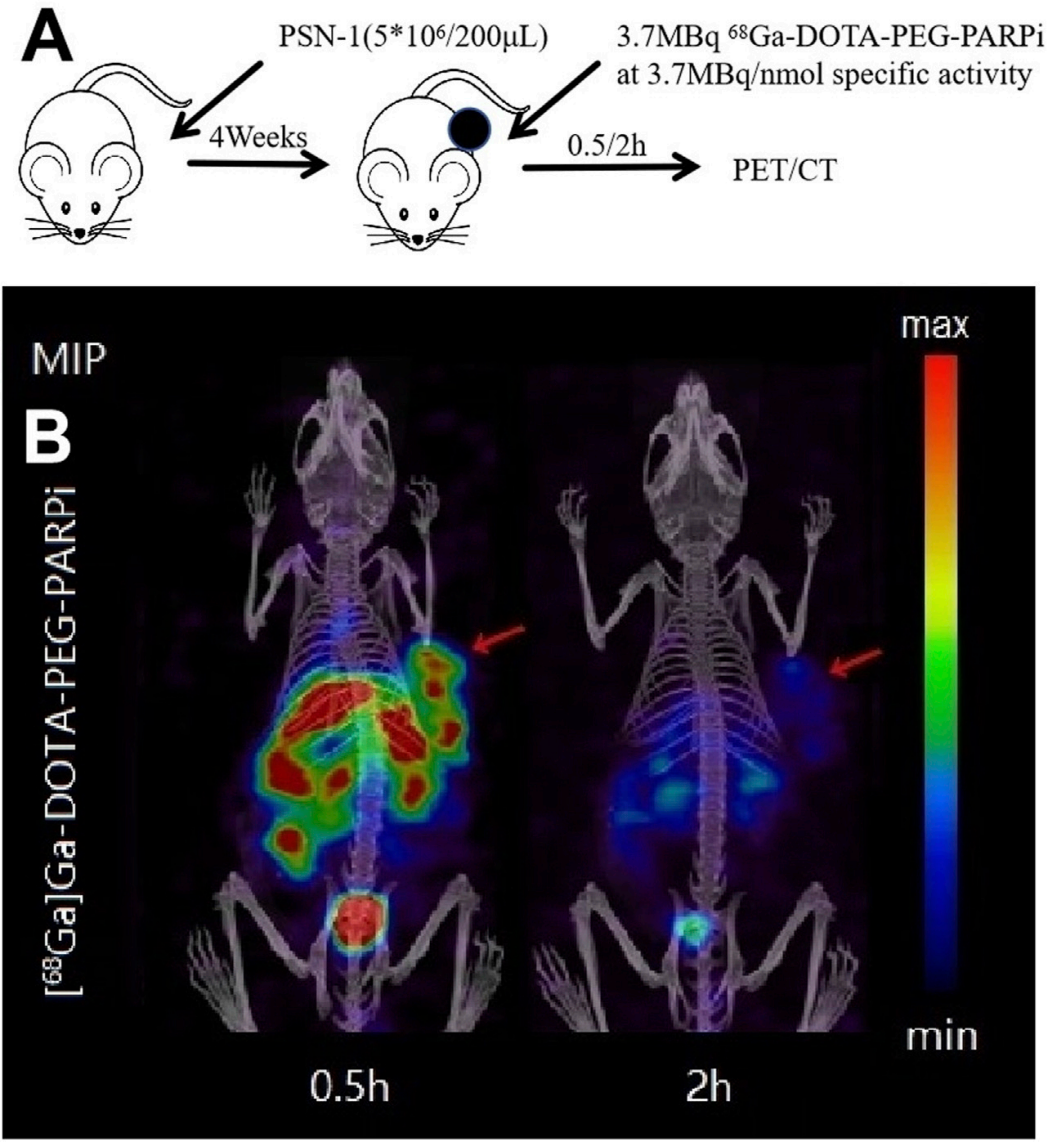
PET/CT result of ^68^Ga-DOTA-PEG-PARPi in PSN-1 models. Molecule imaging of ^68^Ga-DOTA-PEG-PARPi (A). Micro PET/CT images of ^68^Ga-DOTA-PEG-PARPi in PSN-1 models at 0.5, 1, and 2 h after administration (B).
